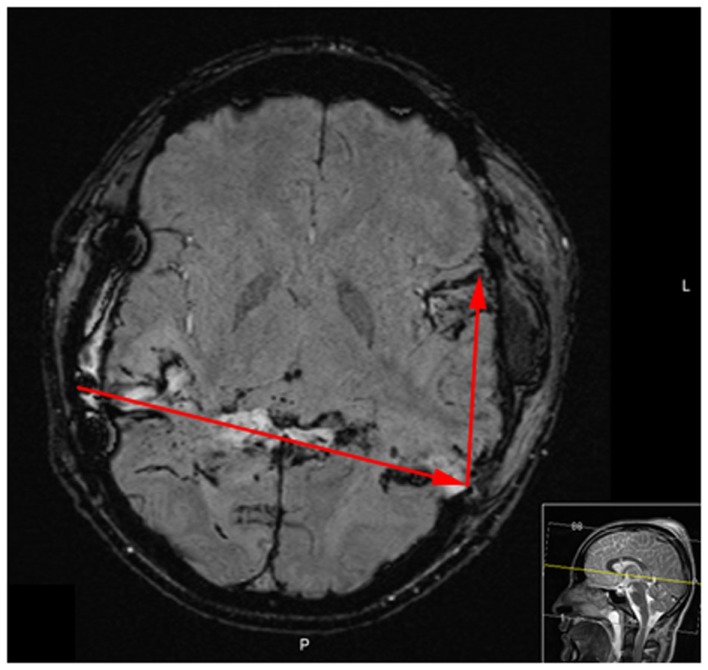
MRI performed [susceptibility weighted imaging protocol (SWI)] on day 16 after injury reveals bilateral temporal damage and hemorrhage along the bullet, yet no signs of ischemic injury.
